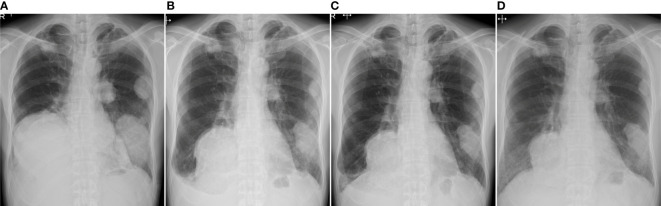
Radiography examination before treatment (A), 1 month (B), 3 months (C), and 18 months (D) after the initial treatment of denosumab. The pulmonary lesions show response to the treatment of denosumab.
